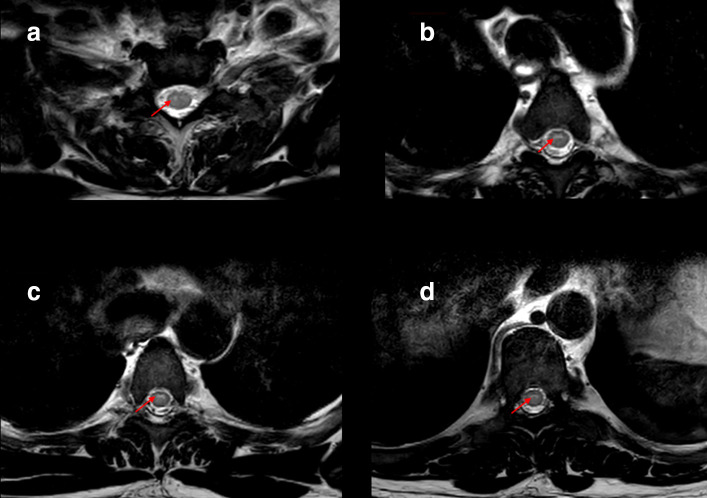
Axial *T*2 weighted images MRI at the level of upper thoracic cord (a, b) and at the level of mid thoracic cord (c, d) show hyperintensities in the ventral horns (arrows) of upper and mid thoracic cord bilaterally.
